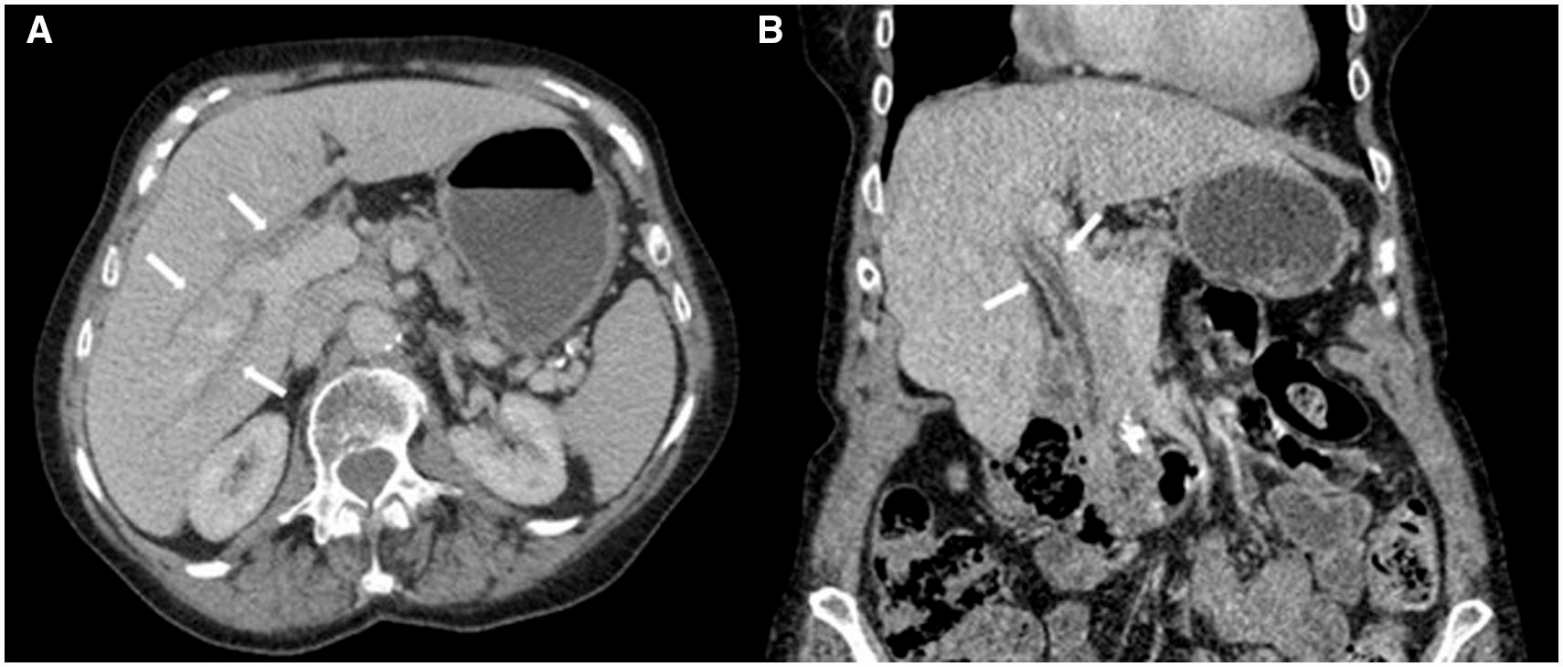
CT performed in September 2020: (A) Axial contrast-enhanced CT image showing mild intrahepatic biliary dilatation with enhancing diffuse ductal wall thickening (white arrows). (B) Coronal reformatted image showing mildly dilated common bile duct with enhancing thickened wall (white arrows).
